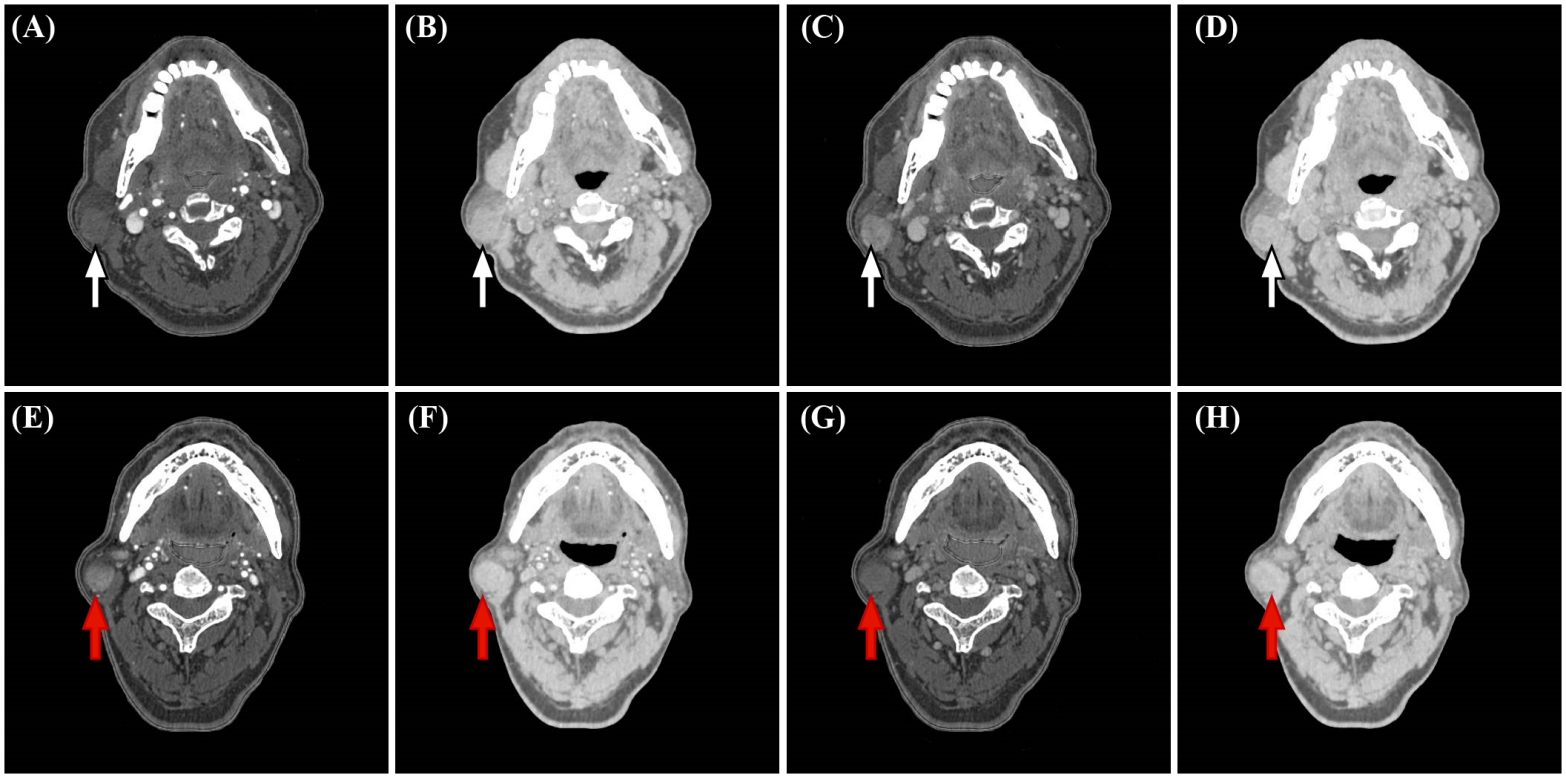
Representative dual-energy CT (DECT) material decomposition (MD) images. (white arrows, A-D) PA in a 67-year-old woman. (red arrows, E-H) WT in a 71-year-old man. (A, E) Iodine-based MD images in the arterial phase. (B, F) Water-based MD images in the arterial phase. (C, G) Iodine-based MD images in the venous phase. (D, H). Water-based MD images in the venous phase.
